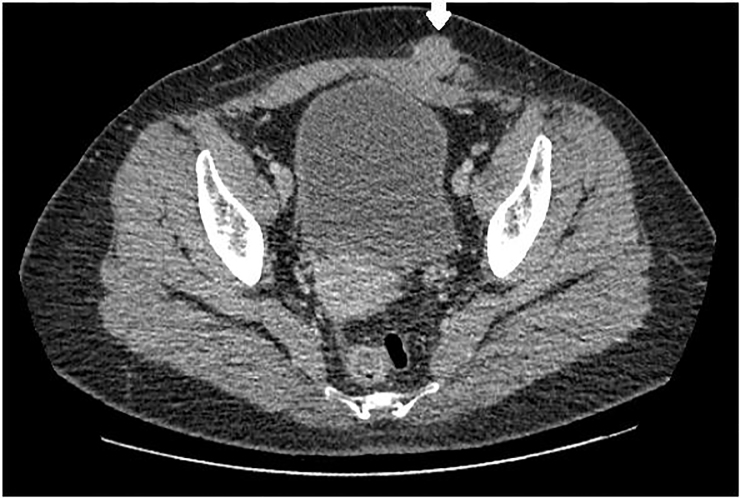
Computed tomography abdomen of the mass in axial plane demonstrating enhancing soft-tissue mass with irregular margins (arrow).
